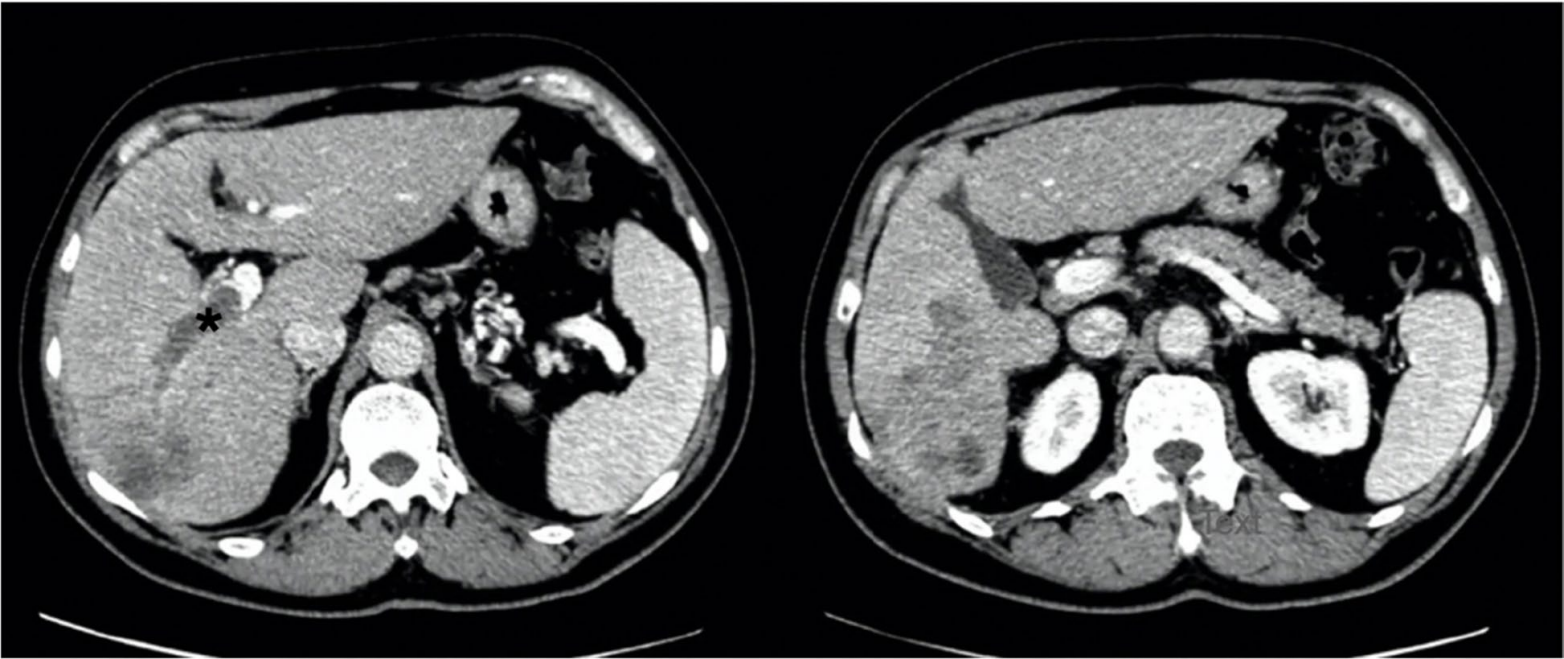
Computed tomography of the cirrhotic patient with combined hepatocellular-cholangiocarcinoma. (*) Tumor thrombus in the right portal vein branch
